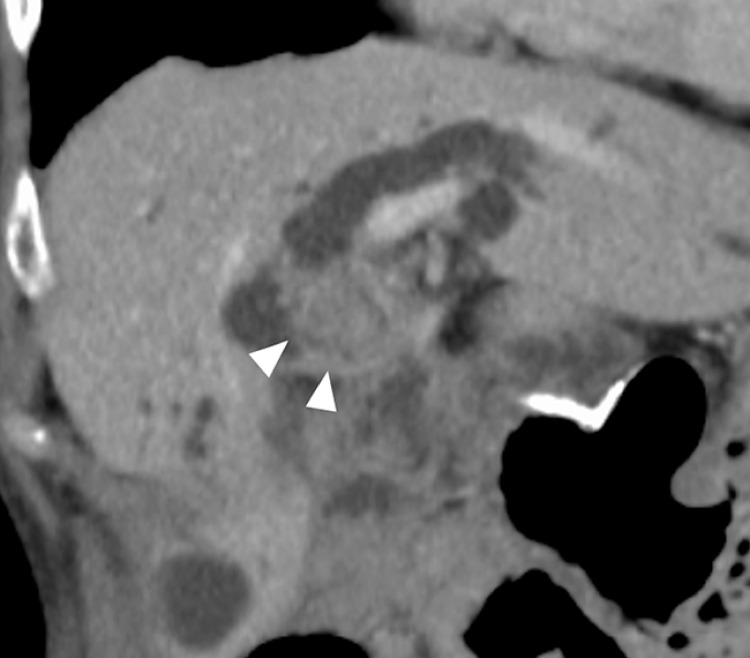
Computed tomography in a 73-year-old patient with Roux-en-Y anatomy showed a 20-mm choledocholithiasis in the hilar bile duct (white arrowheads).
